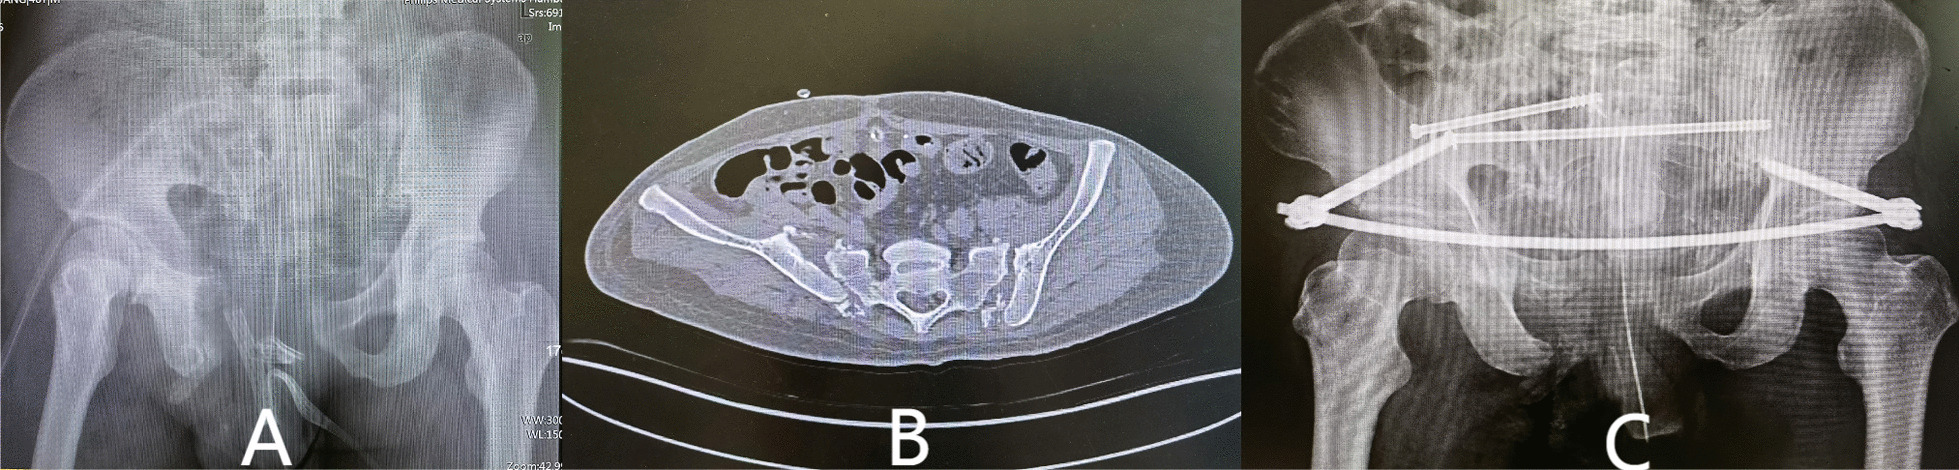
Case 6. A 46-year-old man who was injured from compression underwent S1 sacroiliac screw and S2 transiliac–transsacral screw fixation for a 301SPD type IIIa fracture. A Preoperative X-ray shows bilateral sacroiliac joint injury. B Preoperative CT scan cross-sectional image shows displacement of fracture. C The X-ray image shows that the fracture was fixed satisfactorily
